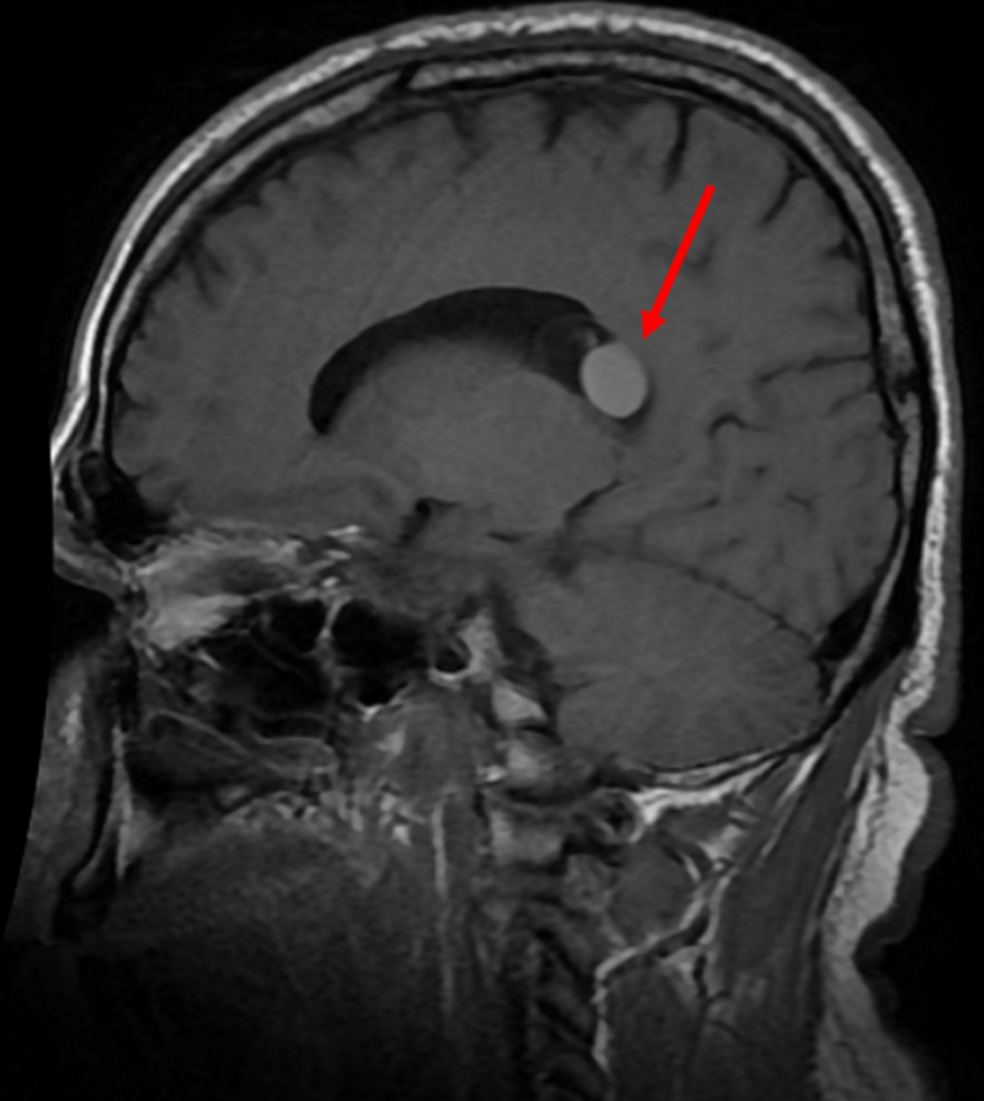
Sagittal T1-weighted brain MRI demonstrating non-enhancing hyperintense lesion.
